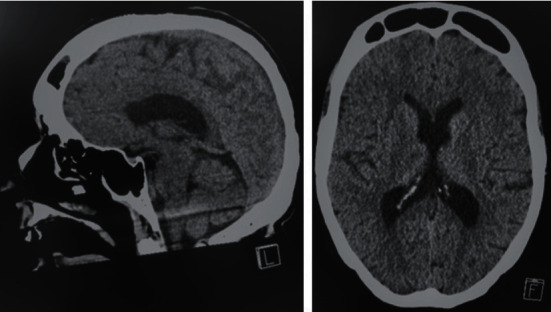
CT brain image obtained in the emergency department indicating a diffuse cerebral edema consistent with meningoencephalitis.
